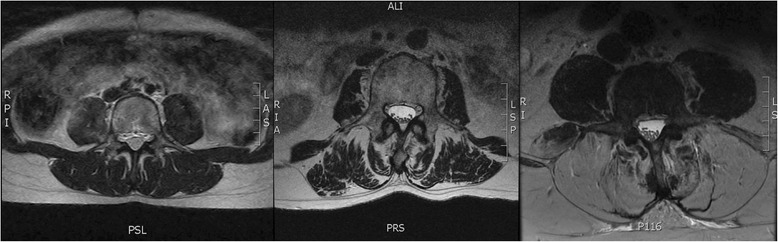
T2 weighted axial MR images of the lumbar spine. Images represent Kjaer grade [16] 0 (left), 1 (center), and 2 (right) muscles of the lumbar spine. All images are from patients undergoing surgery for low back pain related pathology
